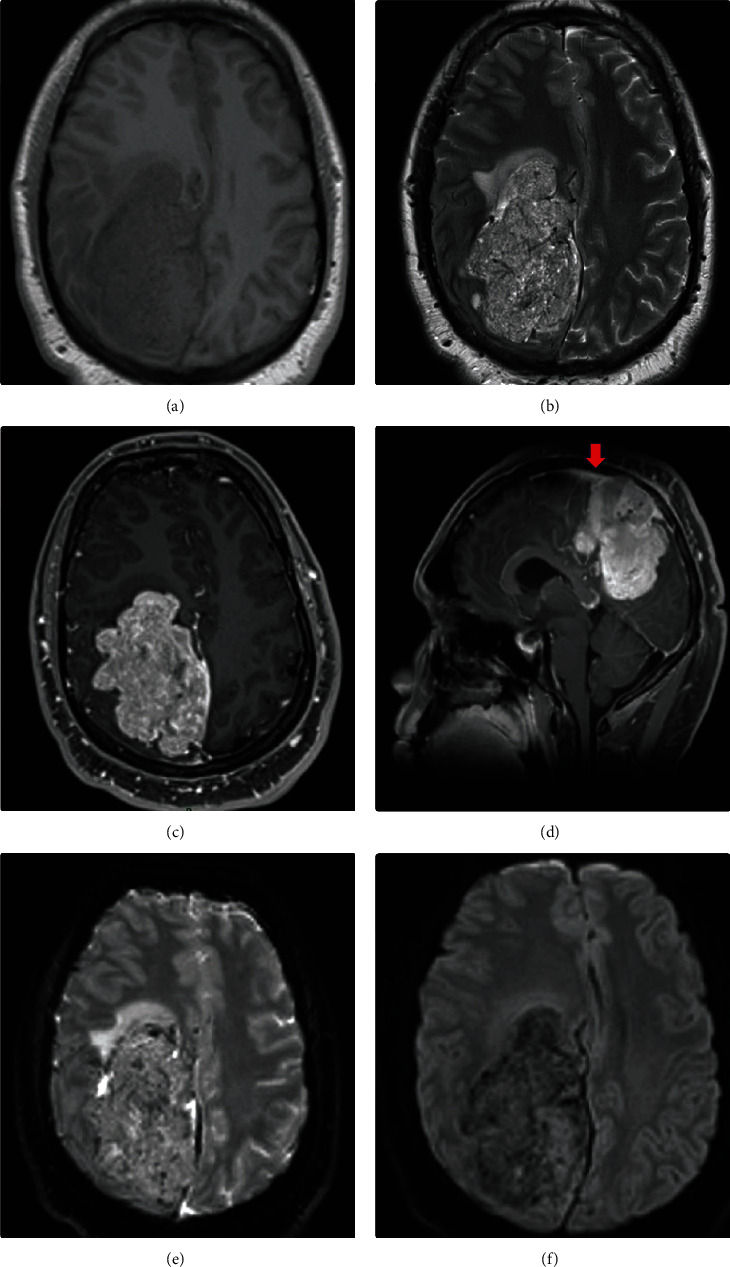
MRI images of a 22-year-old Asian male, presenting with blurred vision, headache, tinnitus, numbness of face, and left hemiplegia. (a) A large macrolobulated extra-axial mass arising from the dura in the supratentorial convexity. The mass has an intermediate signal similar to the brain on T1. (b) The mass demonstrates a heterogeneous “yin yang” appearance of low and high signal intensity on T2. (c) Diffuse contrast enhancement is seen and a (d) dural tail (red arrow) is present. (e, f) Areas of restricted diffusion are seen on diffusion-weighted imaging (DWI).
